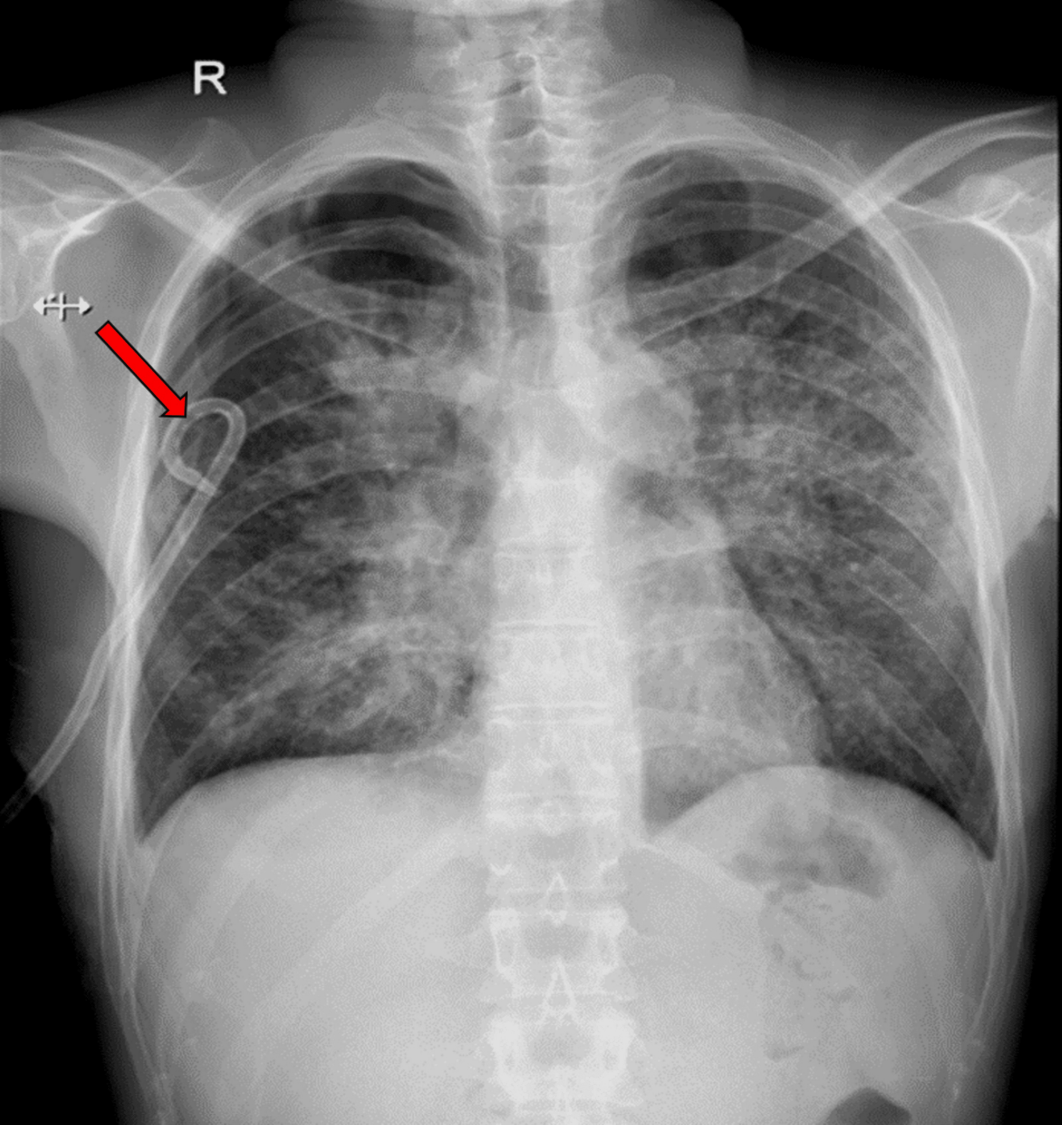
Plain chest radiograph showing right-sided pigtail in situ (red arrow) for draining pleural effusion.
